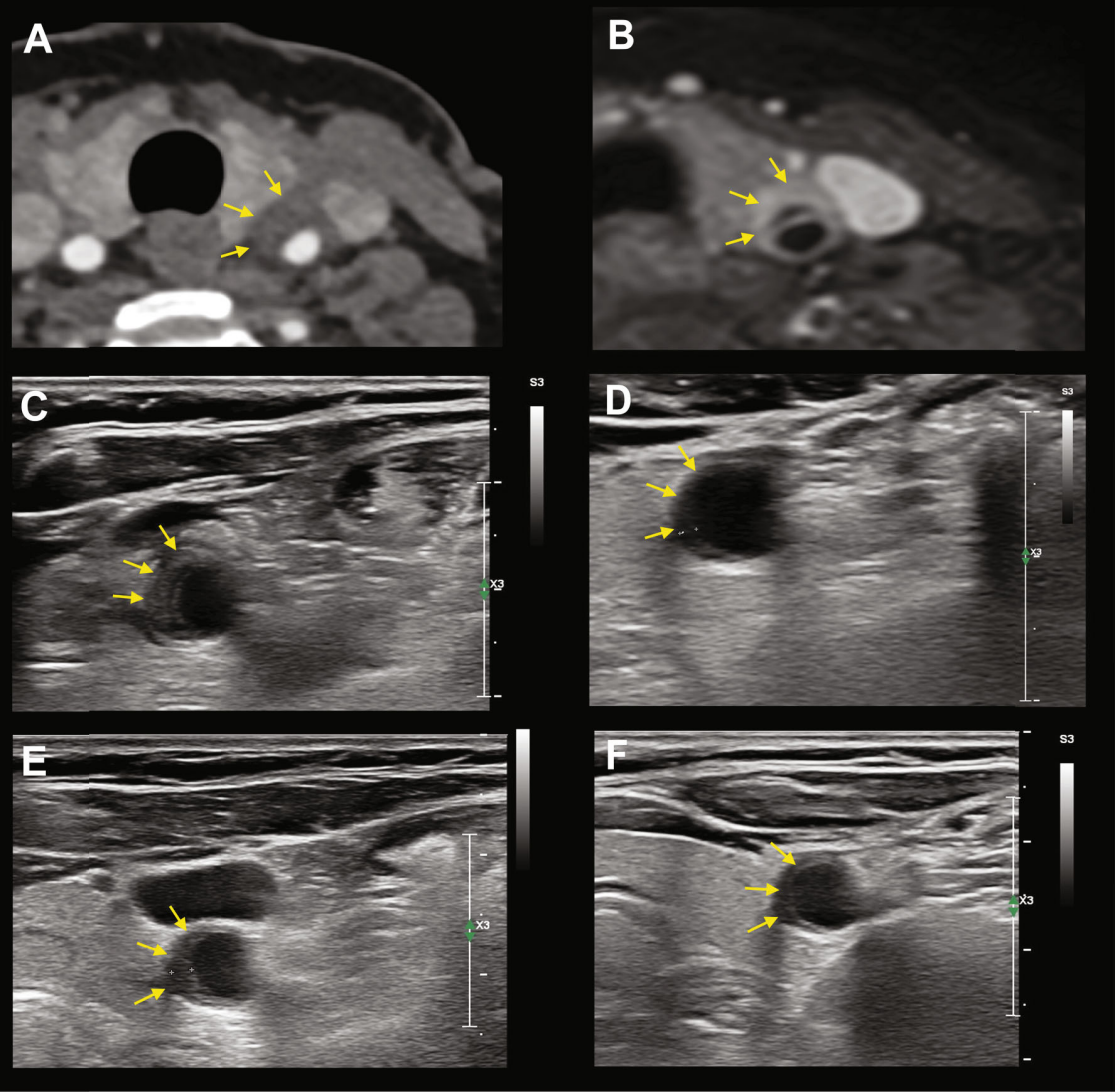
Imaging results. (a) The initial CT showing a perivascular soft tissue alteration due to inflammation and/or edema with slight luminal compression on axial slices. (b) MRI demonstrated a membranous structure within the CCA and a distinct ventrally accentuated circumjacent contrast enhancement on axial T1w fs images. (c) Initial ultrasound imaging with 18 MHz probe (Philips Epiq 5©). Axial imaging of the left common carotid artery in its middle segment, note the multilayered structure (arrows; maximum thickness 3.8 mm in axial plan, longitudinal extension 30 mm). (d) Follow-up imaging after 3 months showing regression of the eccentric vessel wall swelling (arrows; thickness in axial plan 1.1 mm). (e) Follow-up imaging after 6 months showing mildly relapsing wall swelling without a clear multilayered structure (arrows; thickness 2.5 mm). (f) Follow-up imaging after 9 months showing regression of the vessel wall thickening (thickness 1.4 mm).
